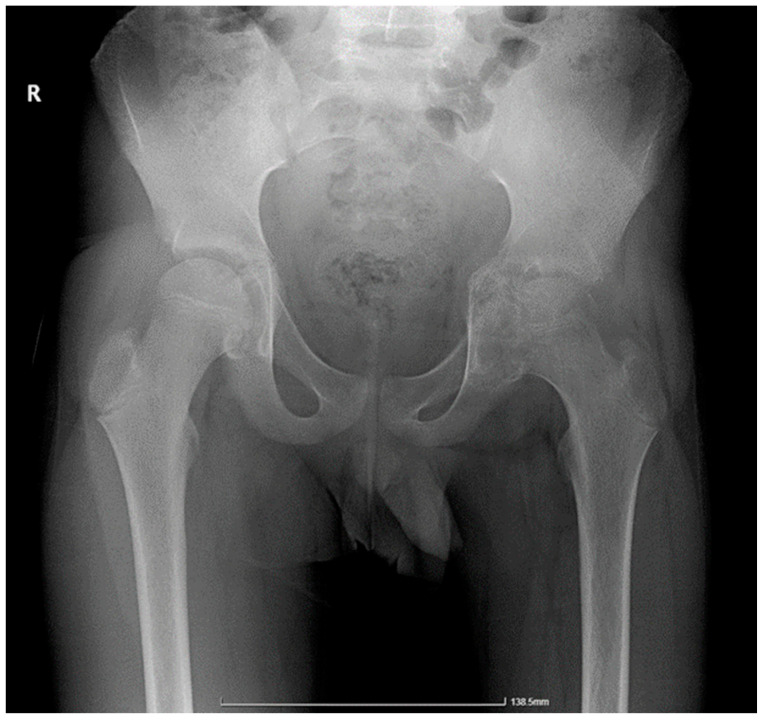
Pelvic radiography: areas of osteolysis at the level of the left hip joint with a decreased joint space, along with thinning and erosion of the joint contours. R indicates the right side of the parient.
